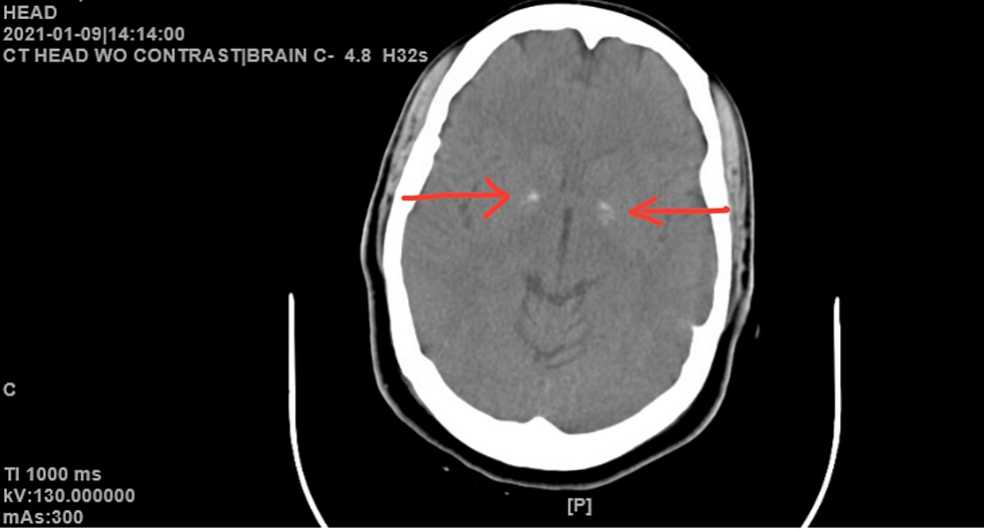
Axial CT without contrast scan of the brain taken in 2021. Red arrows indicate bilateral basal ganglia calcifications that are new in comparison to the 2019 imaging
